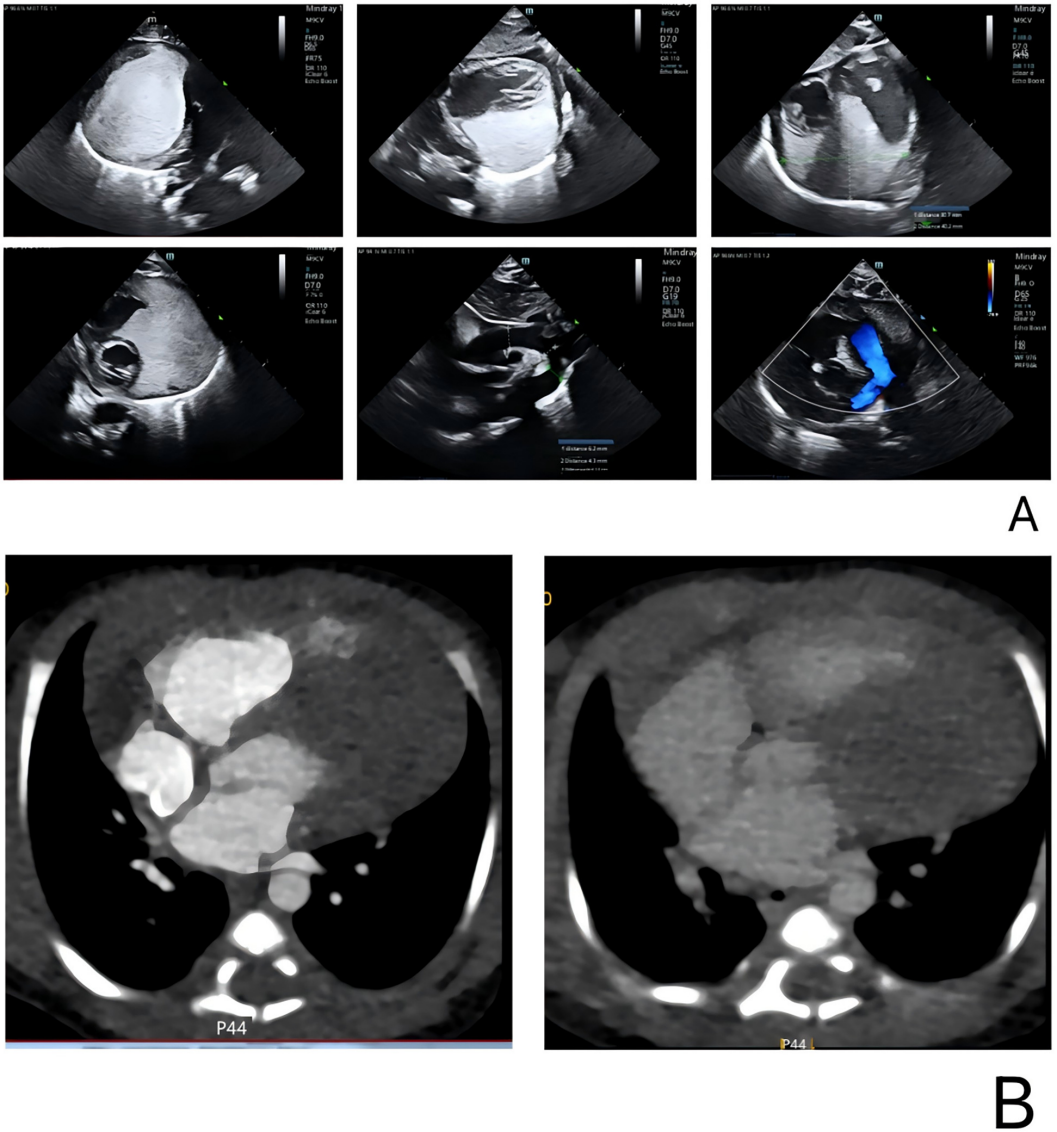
Cardiac color Doppler ultrasound and CT in the pediatric patient. (A) A cardiac ultrasound performed two days after birth revealed a hyperechoic region adjacent to both ventricles, as well as near the aorta and pulmonary artery. The lesion measured 40 × 34 × 36 mm, and no significant blood flow was detected on color Doppler imaging. (B) Cardiac enhanced CT showed a large irregular lesion in the pericardium, left side, clear edge, 62 × 33 mm, plain CT value 61 HU, enhanced scan showed mild homogeneous enhancement. Morphology of great vessels: Normal aorta, left ventricle, branches. Atria: Normal size left and right. Ventricles: Normal size left and right. Valves: Normal shape and density aortic, pulmonary, mitral, tricuspid.
